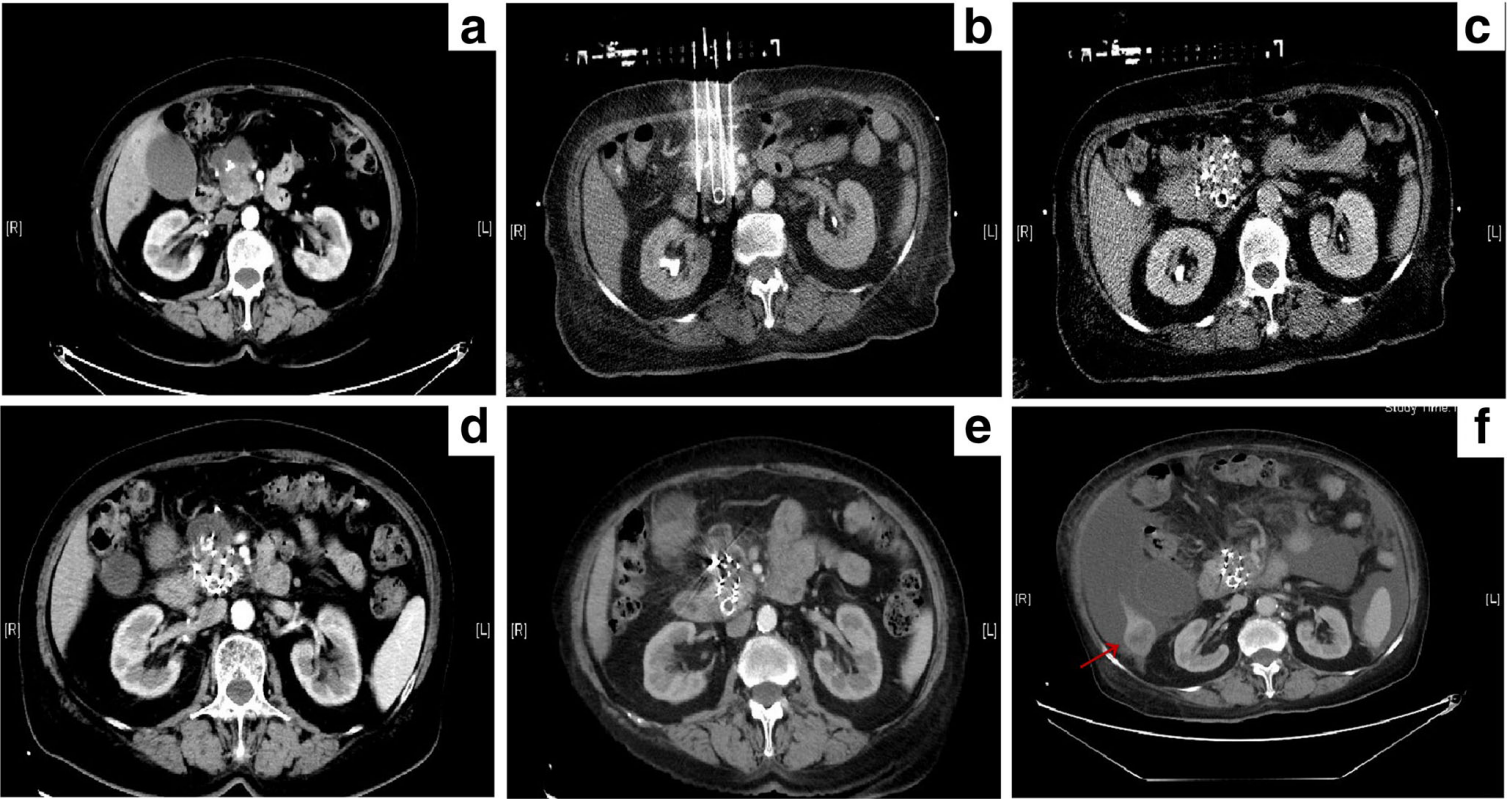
A 75-year-old female with pancreatic cancer at the junction of the body and pancreas head, and the superior mesenteric artery was involved in. a Abdominal CT revealed the tumor before the treatment. b Intraoperative puncture procedure under the CT-guided. c Coverage and the distribution of implanted seeds after brachytherapy. d, e The local lesion achieved complete response (CR) after 2 months (d) and 6 months (e) follow-up. f The patient has survived 17.73 months, and died of cachexia. Though there were liver metastasis (red arrow) and malignant ascites, the local lesion had been still under the control
